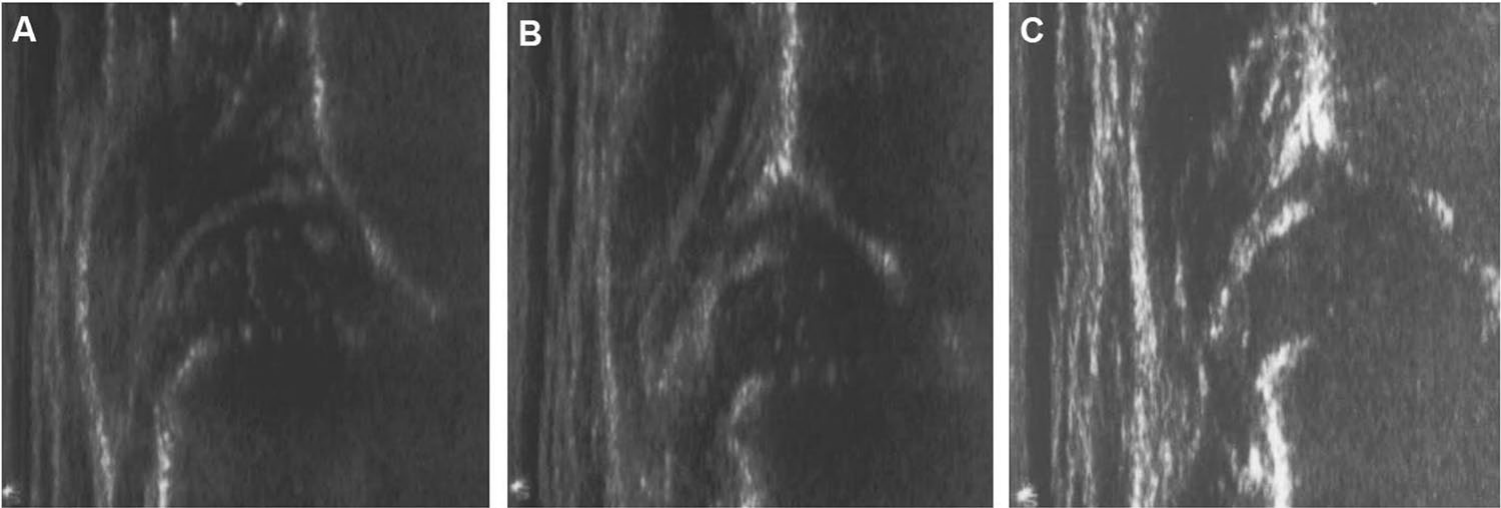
Ultrasound examination of female, right side: A initial ultrasound examination at the age of 41 days with an ultrasound hip type IV and start of treatment with the Tübingen splint, B ultrasound control after 3 weeks showing a centered hip with alpha angle of 46°, C successful treatment after 15 weeks with normal ultrasound characteristics and an alpha angle of 67°
